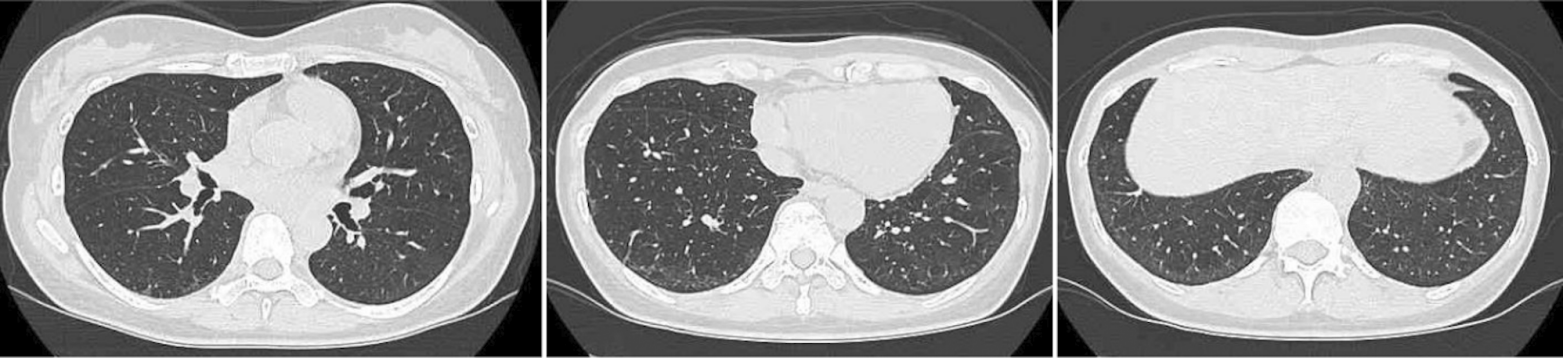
Chest computed tomography 4 months after filgotinib initiation.
